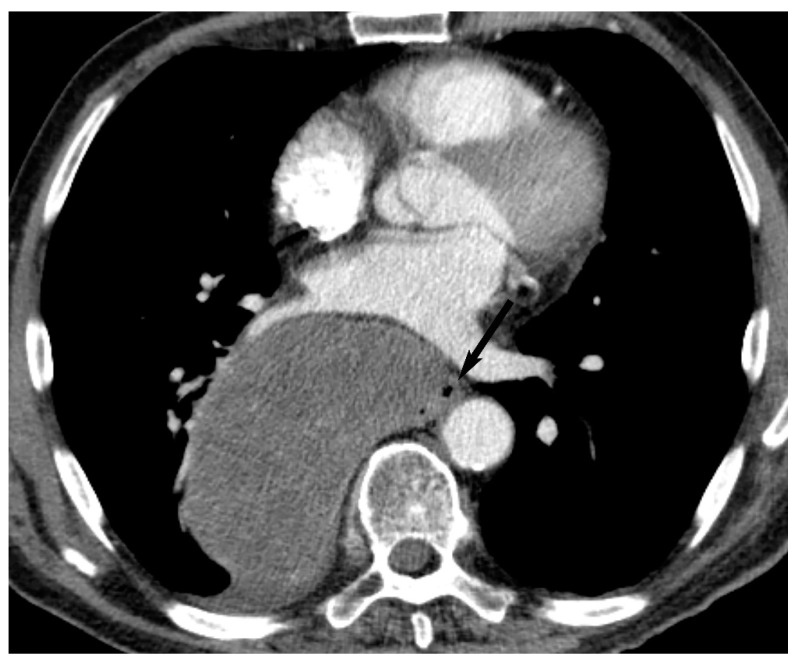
Esophageal gastrointestinal stromal tumor (GIST). CT shows a large heterogeneous mass in the visceral mediastinum inseparable from the esophagus (arrow). Biopsy showed gastrointestinal stromal tumor.
